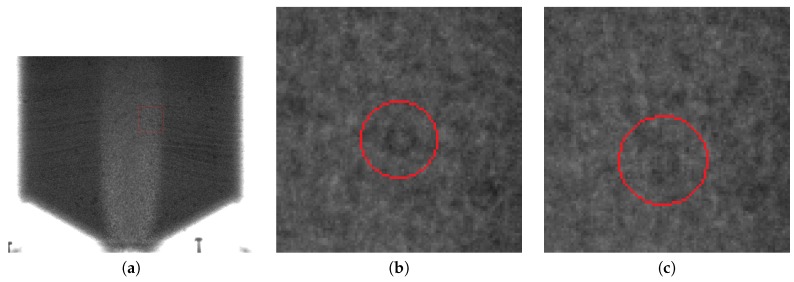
Difficulty of distinguishing markers in radiography images: (a) frame with the investigated area marked, (b) zoomed-in marker, and (c) the same marker on the next frame.
